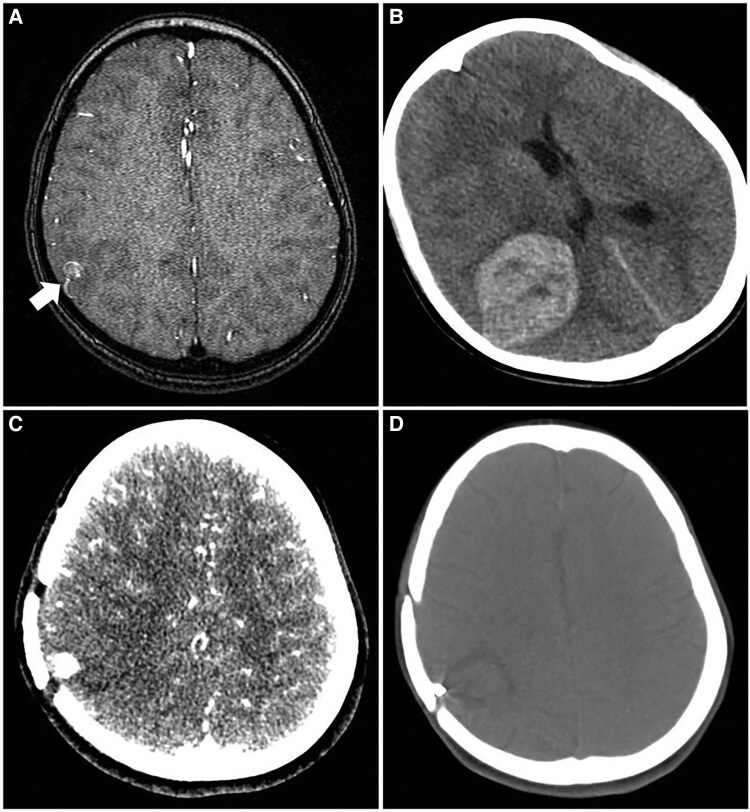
Index cerebral magnetic resonance angiography on admission (*A*, intracranial infectious aneurysm indicated by white arrow); computed tomographic angiography performed at the time of intracranial haemorrhaging (*B*), 1 month after craniotomy (*C*) and before discharge (*D*), respectively.
